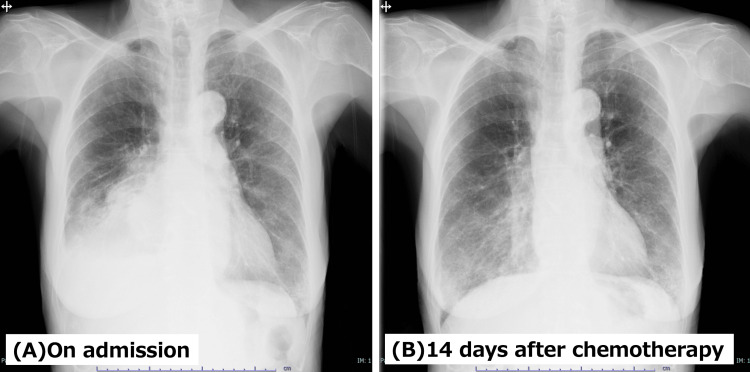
Serial chest radiographs before and after chemotherapy. (A) Chest radiograph on admission shows a large right pleural effusion with associated right basal atelectasis. (B) Chest radiograph 14 days after initiation of chemotherapy demonstrates a marked reduction in the right pleural effusion with improved right lung expansion.
